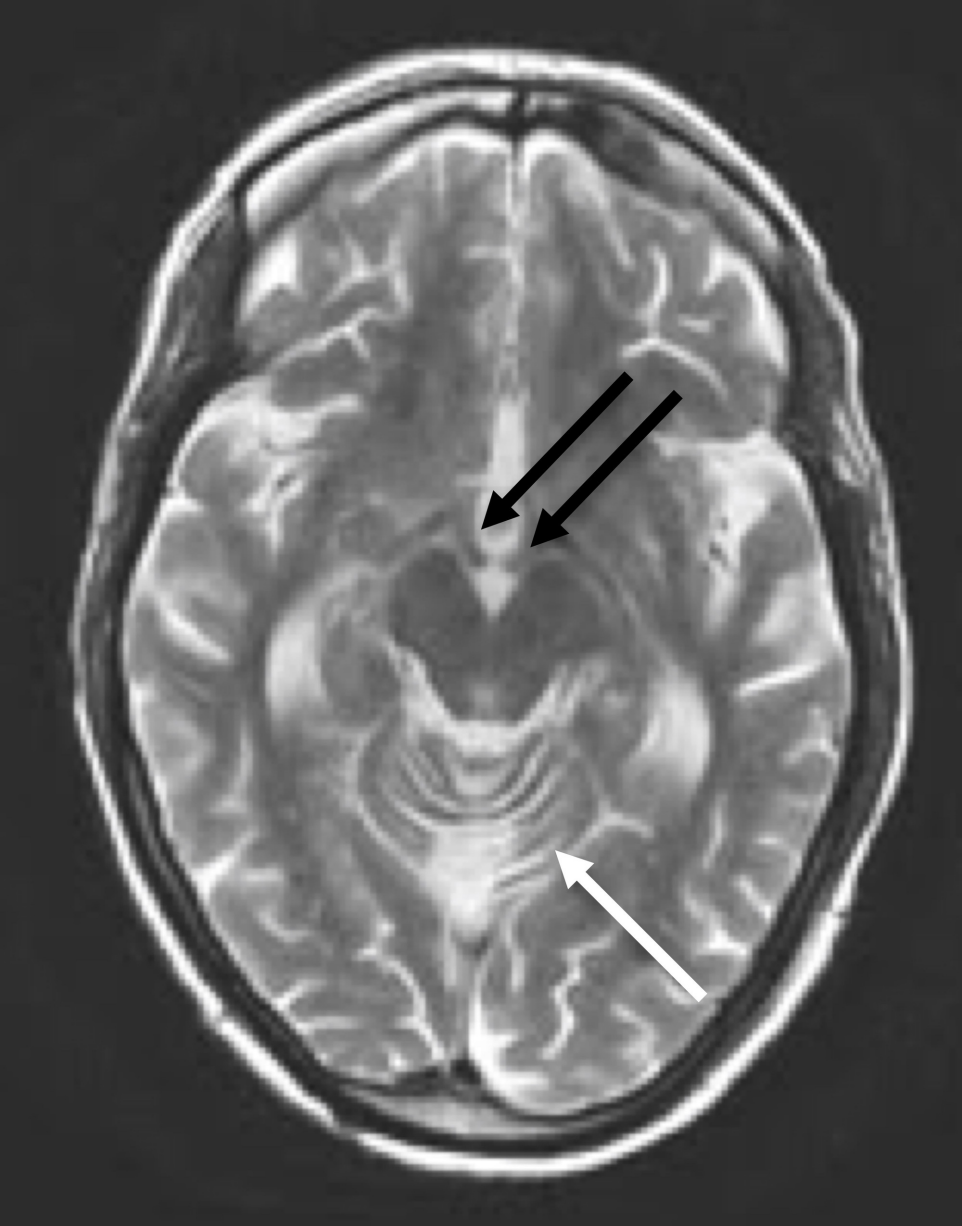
Axial T2-weighted MRI of the head Axial T2-weighted MRI of the head at the level of the midbrain shows prominent vermian folia and abnormal increased signal in the superior vermis (white arrow) as well as normal size and signal intensity of the mamillary bodies (black arrows)
